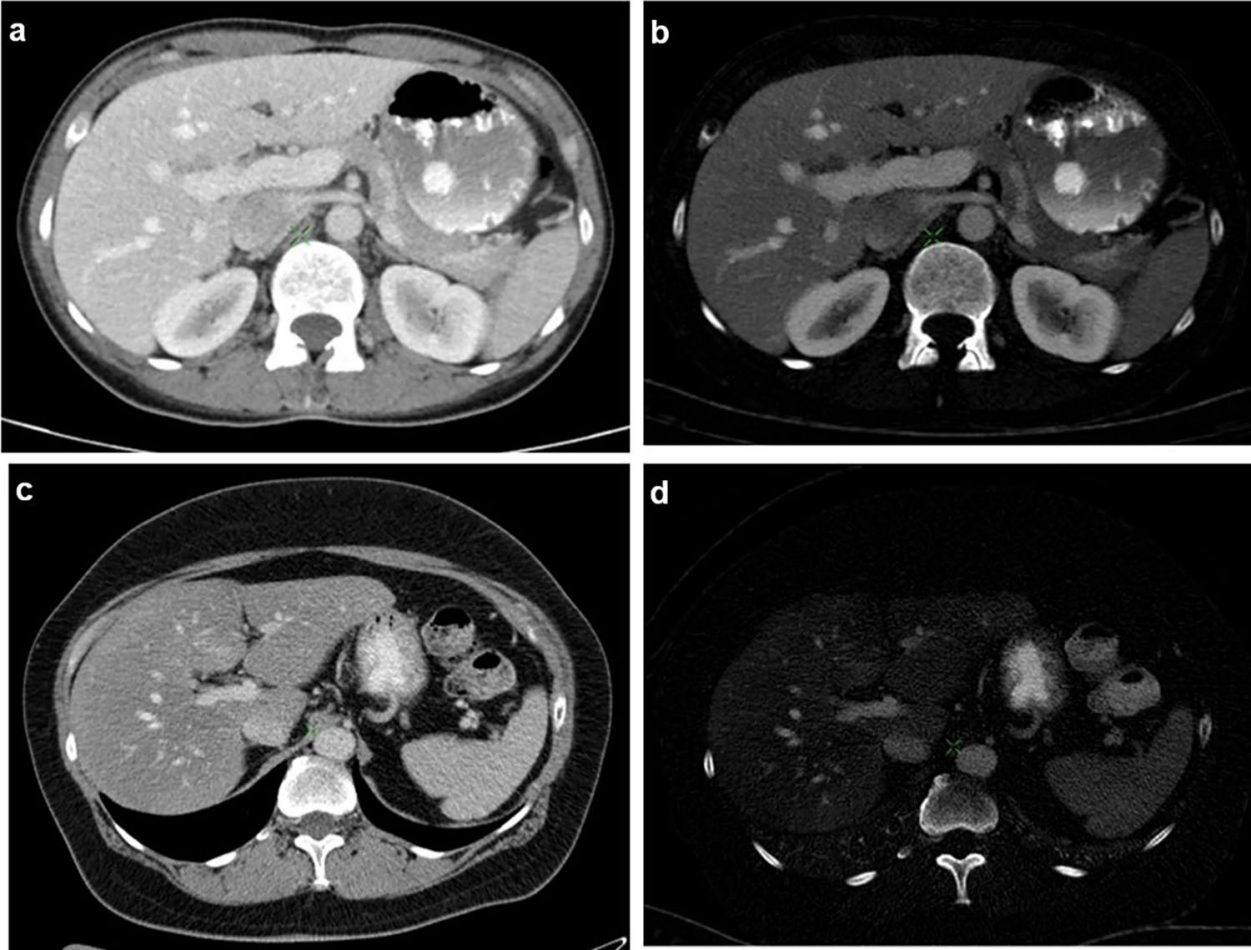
Portal venous phase DECT images of the upper abdomen in transverse plane. a, c One hundred twenty-kilovoltage peak HU images. b, d Material density iodine concentration images. The images in the upper row present the liver parenchyma of a 52-year-old male, healthy patient (CT value: 111 HU; iodine value: 2.75 mg/ml). In the lower row, DECT of the liver of a 51-year-old male patient with hepatic steatosis is shown, with lower CT value (68 HU) and decreased iodine value (0.88 mg/ml)
